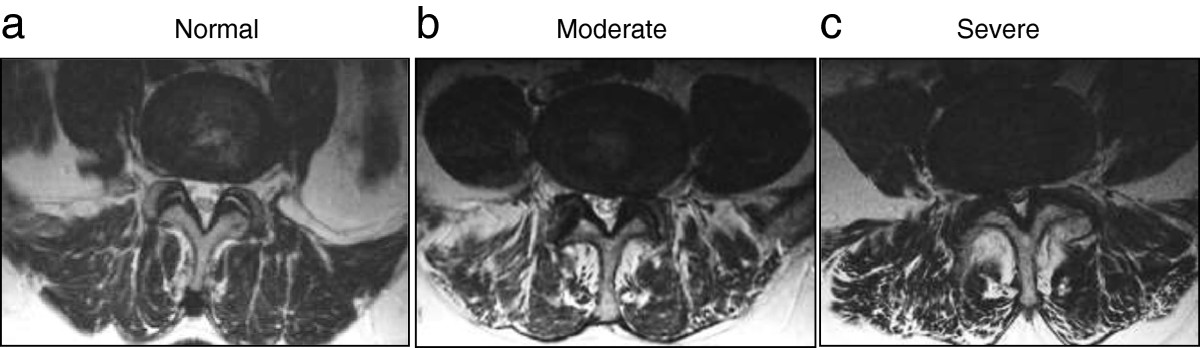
Axial T2-weighted model images of representative cases that were used to grade central spinal canal in visual assessment. a) normal central spinal canal; b) moderate central spinal canal stenosis; c) severe central spinal canal stenosis.
